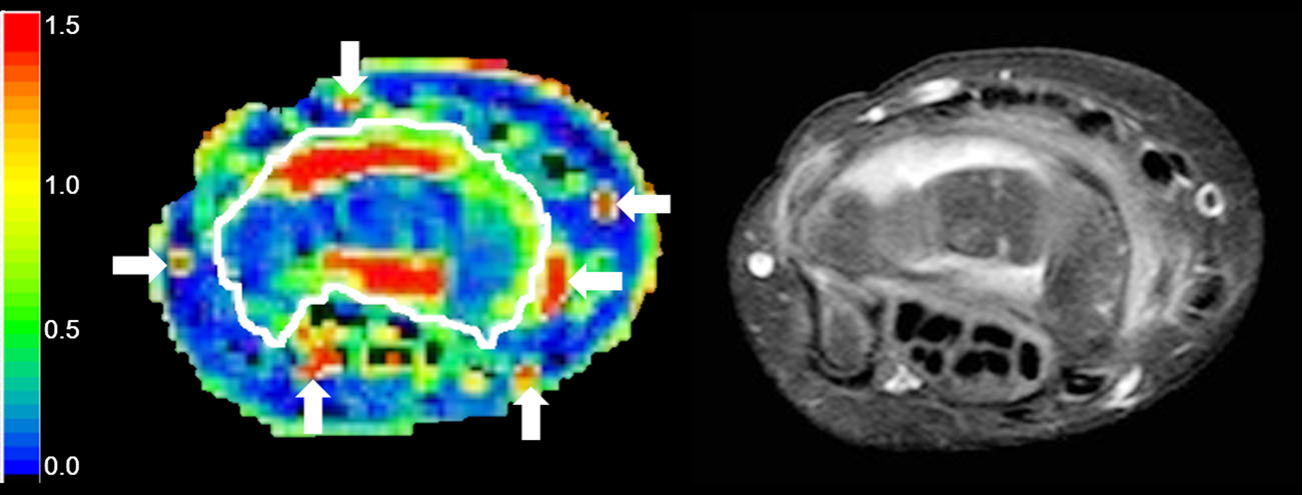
Example of a manually drawn region of interest (*white line*) on the maximal enhancement map (*left*) based on axial wrist MRI in a 9-year-old female with clinically active juvenile idiopathic arthritis. The *arrows* indicate the arteries and vessels, which have not been included in the region of interest. A high spatial resolution T1-weighted image with fat saturation after intravenous contrast administration (*right*) is added for anatomical reference
